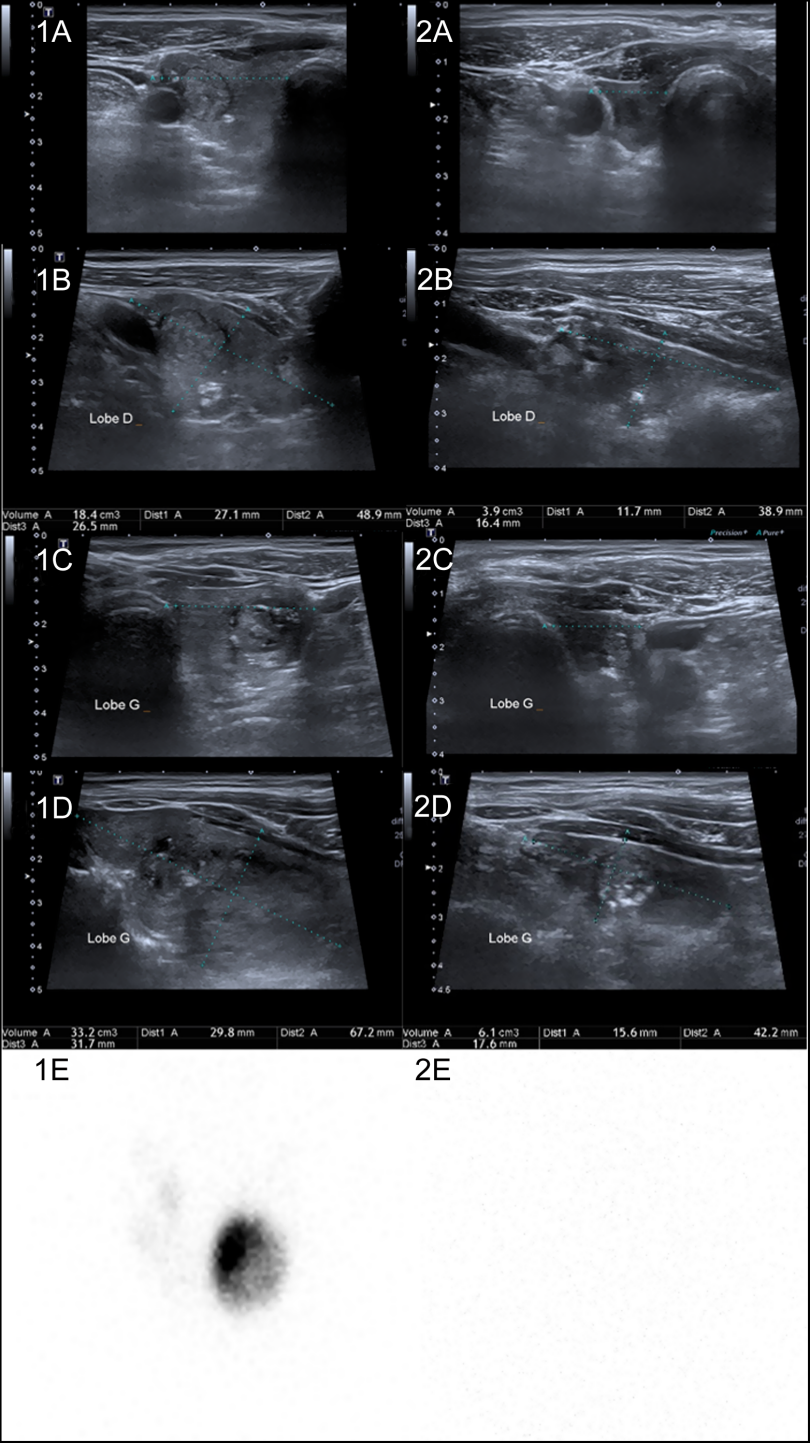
Thyroid ultrasound and ^123^I-scintigraphy imaging before (left column) and after (right column) ICI therapy. (1a and 1b) Transverse and longitudinal US images of the right thyroid lobe before ICI therapy, assessing a 18.4 mL volume and three nodules. (1c and 1d) Transverse and longitudinal US images of the left thyroid lobe before ICI therapy, assessing a 33.2 mL volume and two nodules. (2a and 2b) Transverse and longitudinal US images of the right thyroid lobe 7 months after ICI therapy initiation, assessing a 3.9 mL volume and no visible nodule (except for an isolated macrocalcification). (2c and 2d) Transverse and longitudinal US images of the left thyroid lobe 7 months after ICI therapy initiation, assessing a 6.1 mL volume and only one visible nodule. (1e) ^123^I-scintigraphy 13 days after the first ICI infusion showing a left lobe heterogeneous hot nodule and suppressed uptake in the rest of the thyroid. (2e) ^123^I-scintigraphy 7 months after ICI therapy initiation showing absent ^123^I uptake.
